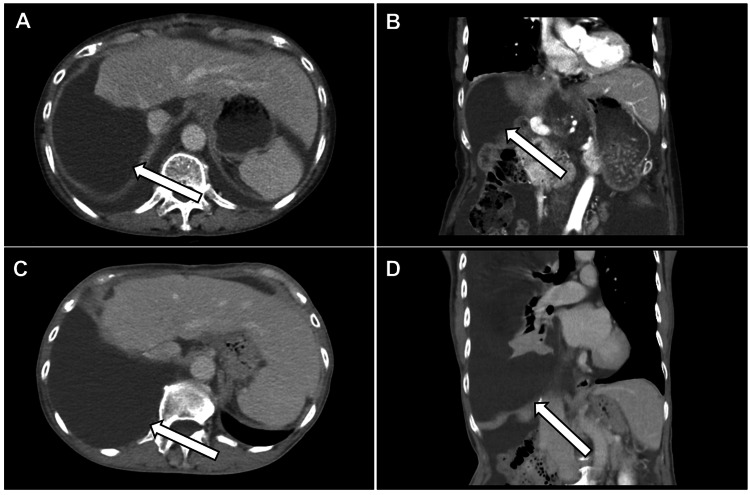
CT images of chylous ascites and chylothorax. (A) Axial and (B) coronal contrast-enhanced CT images showing chylous ascites. (C) Axial and (D) coronal contrast-enhanced CT images showing a chylothorax. Chylous ascites and chylothorax are indicated by white arrows.
